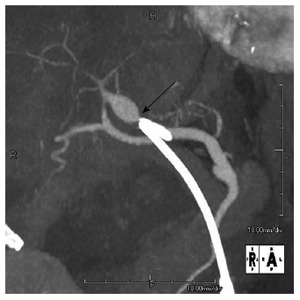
Abdominal computed tomography angiography. A 13 mm × 10 mm pseudoaneurysm was found at the proximal anterior segment of the right hepatic artery; The hepatic side edge of the plastic stent (black arrow) was close to the pseudoaneurysm.
